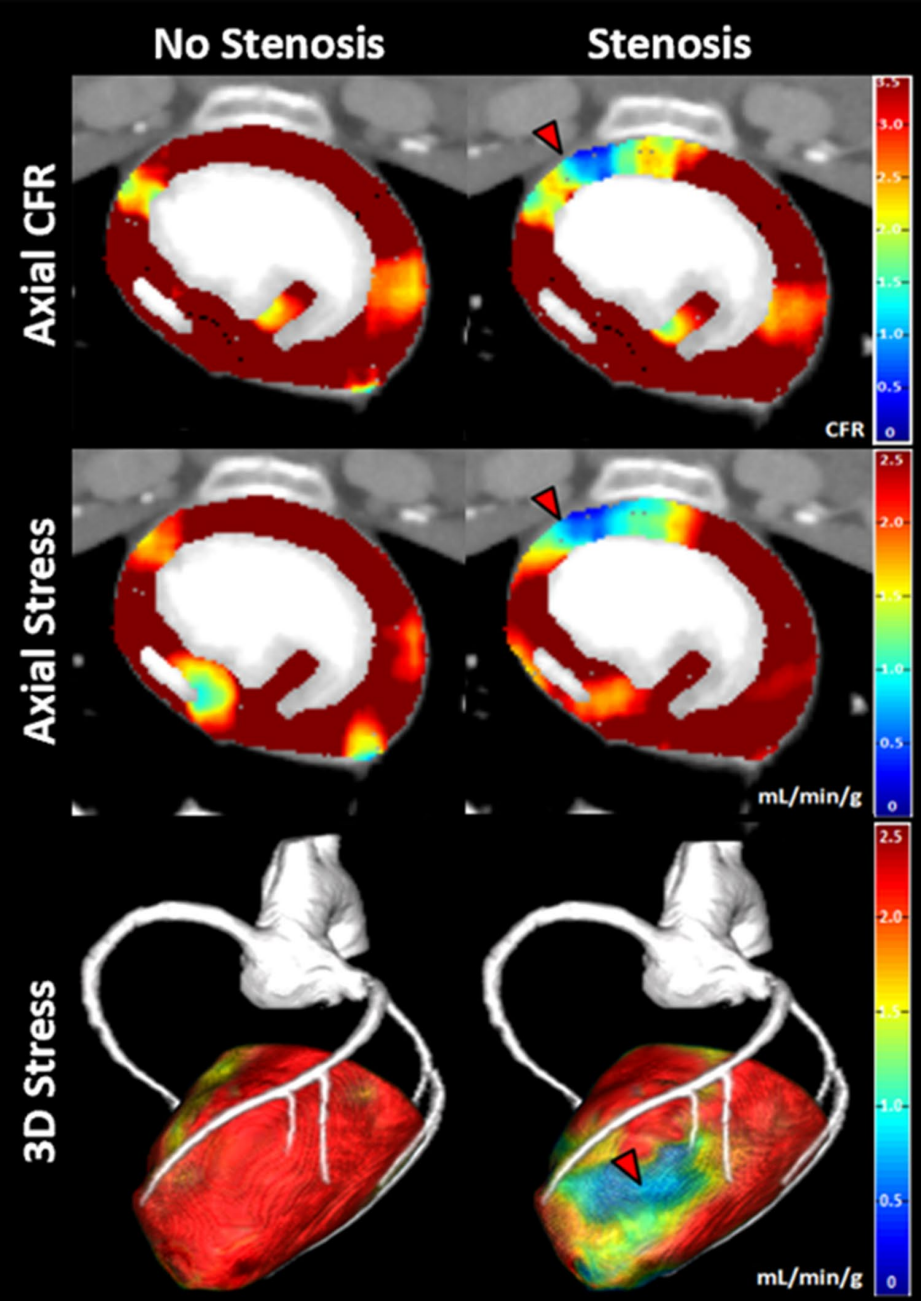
Example visualization of the low-dose cardiac CT technique. Low-dose CFR and stress perfusion in the absence and presence of a significant left anterior descending (LAD) coronary artery balloon stenosis (FFR = 0.70), with co-registered CTA displayed. A LAD perfusion deficit is shown (red arrows). The color bars indicate low-dose CFR and stress perfusion measurement in mL/min/g
